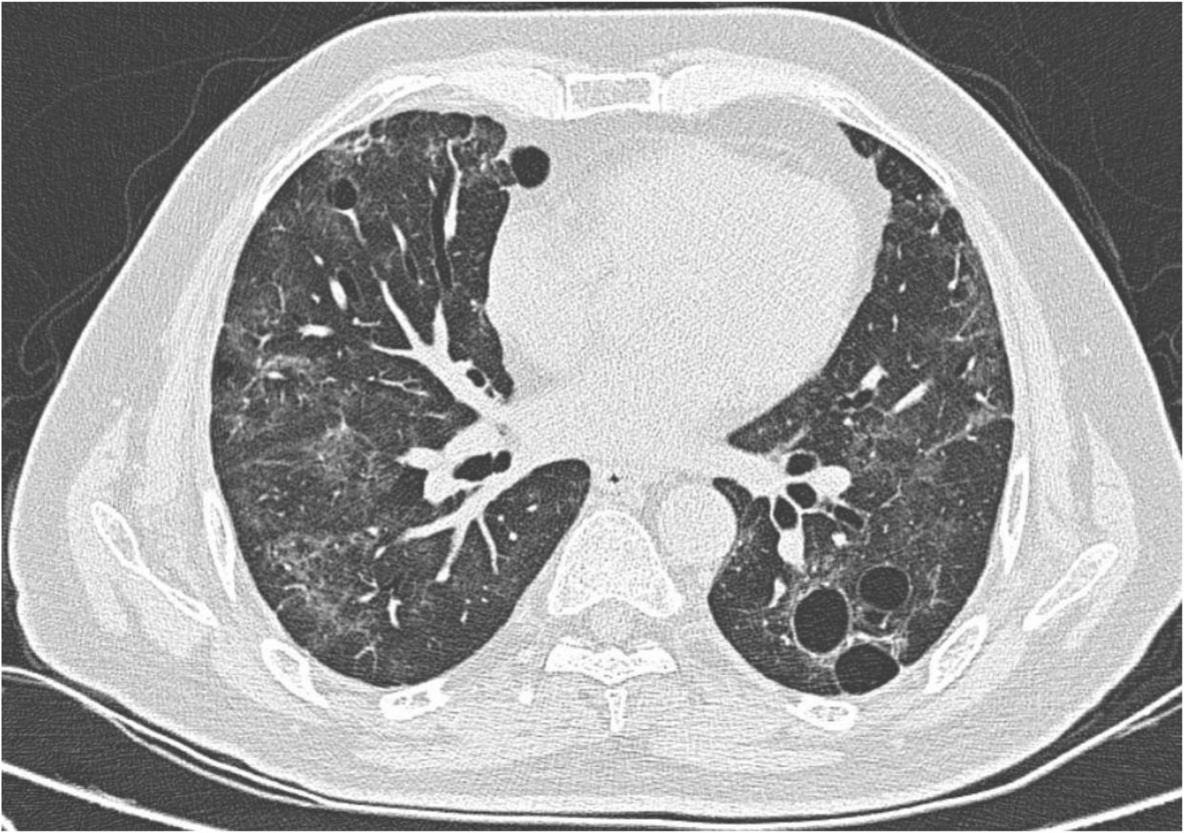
Axial CT thorax of a 60-year-old COVID pneumonia patient showing multiple thin-walled air-filled cystic lesion in the bilateral lower lobes. Areas of GGOs with interlobular septal thickening are also seen
